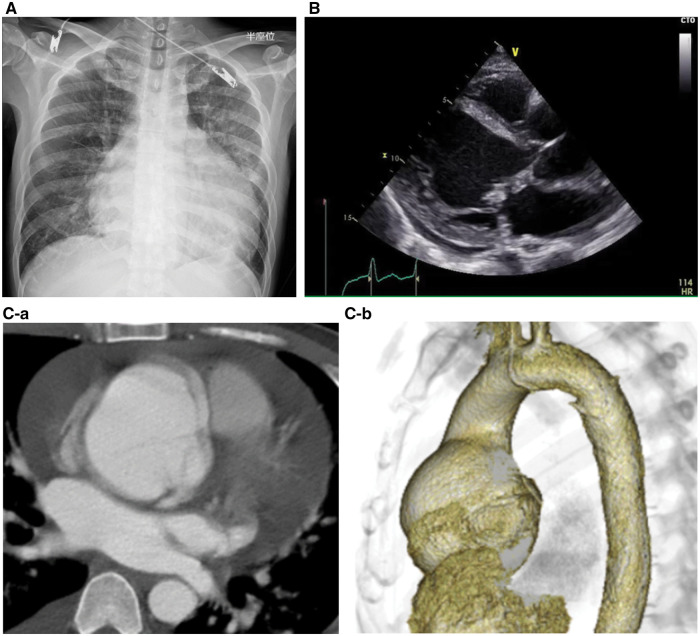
(A) Preoperative chest X-ray. (B) Vegetation on the mitral valve by echocardiography. (C-a and C-b) Dissecting aneurysm of the sinus of Valsalva by computed tomography.
